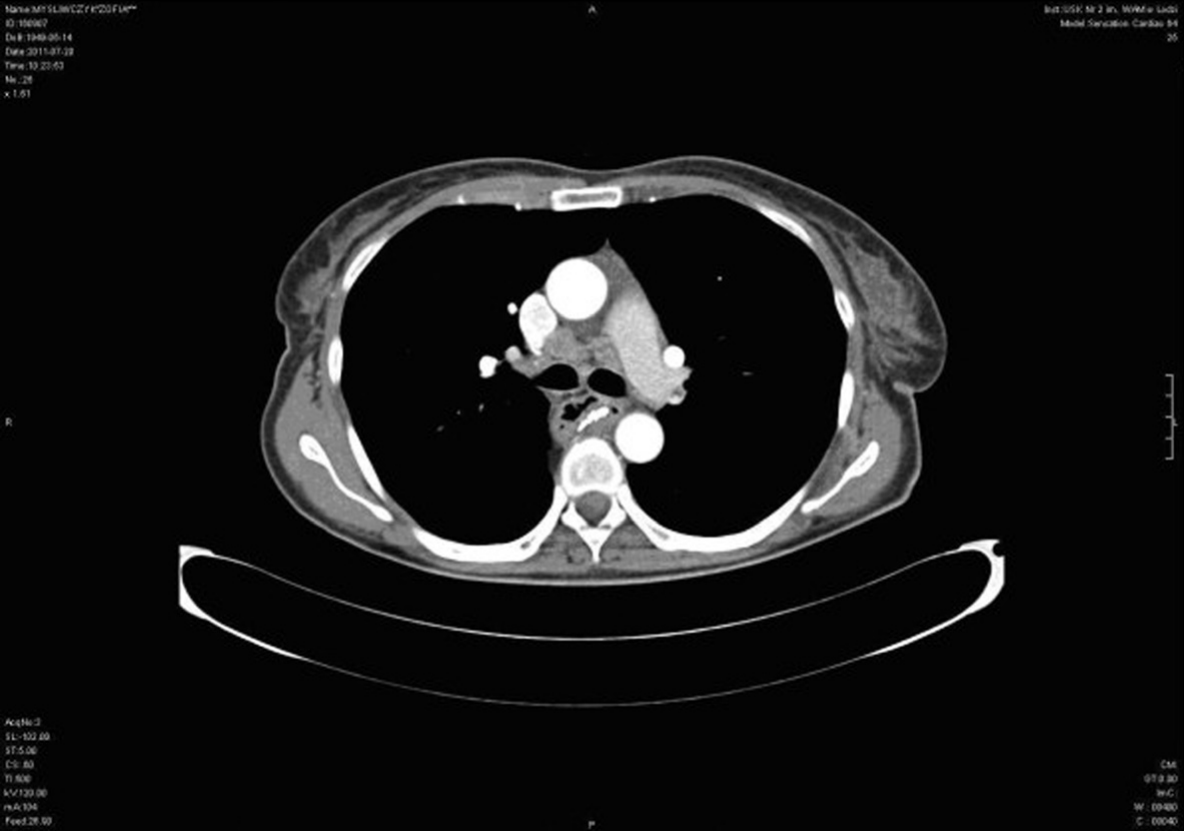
Computed tomography (scan A, B): gastroesophageal anastomosis at the level of tracheal bifurcation, below visible thickening of gastric mucosa.
